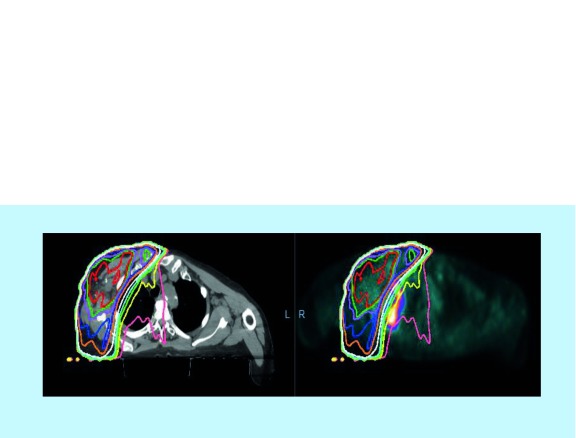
Prior right lateral chest wall mass radiation dosimetry (right) and radiation field overlying the PET/CT scan visualizing the radiation recall pneumonitis (left).
